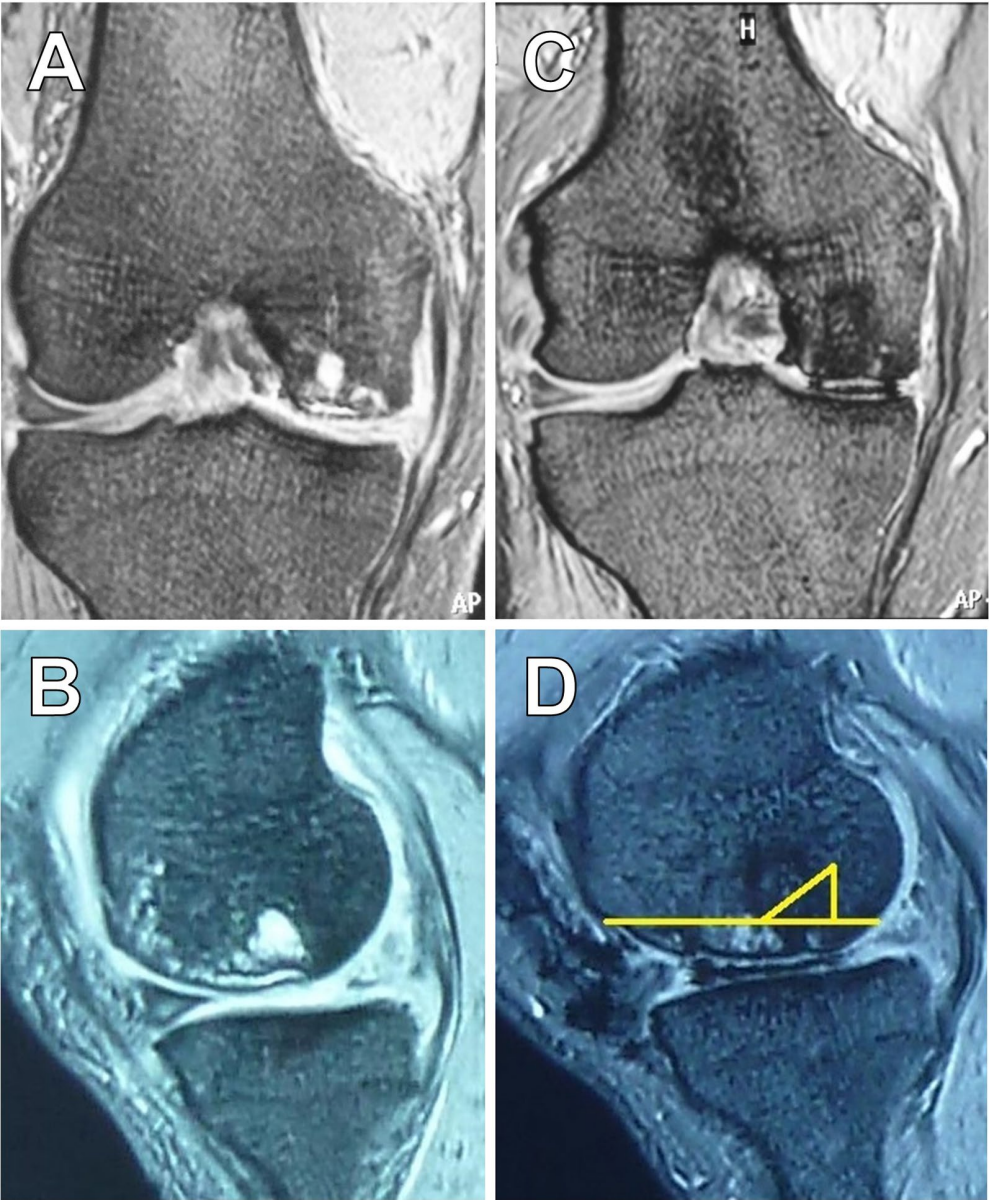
Magnetic resonance image of pre- and post-osteochondral autologous transfer operation. Low intensity on T2-weighted images of the right medial femoral condyle (A, C coronal plane; B, D sagittal plane). The images were taken before osteochondral autologous transfer (A, B) and before total knee arthroplasty (C, D). *Yellow lines* show the resection line of two parts of the tissue
